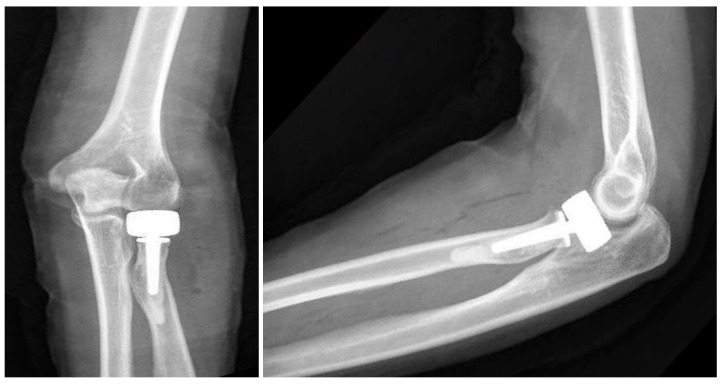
Radiographical AP and LL view of the implanted cemented bipolar radial head prosthesis.
